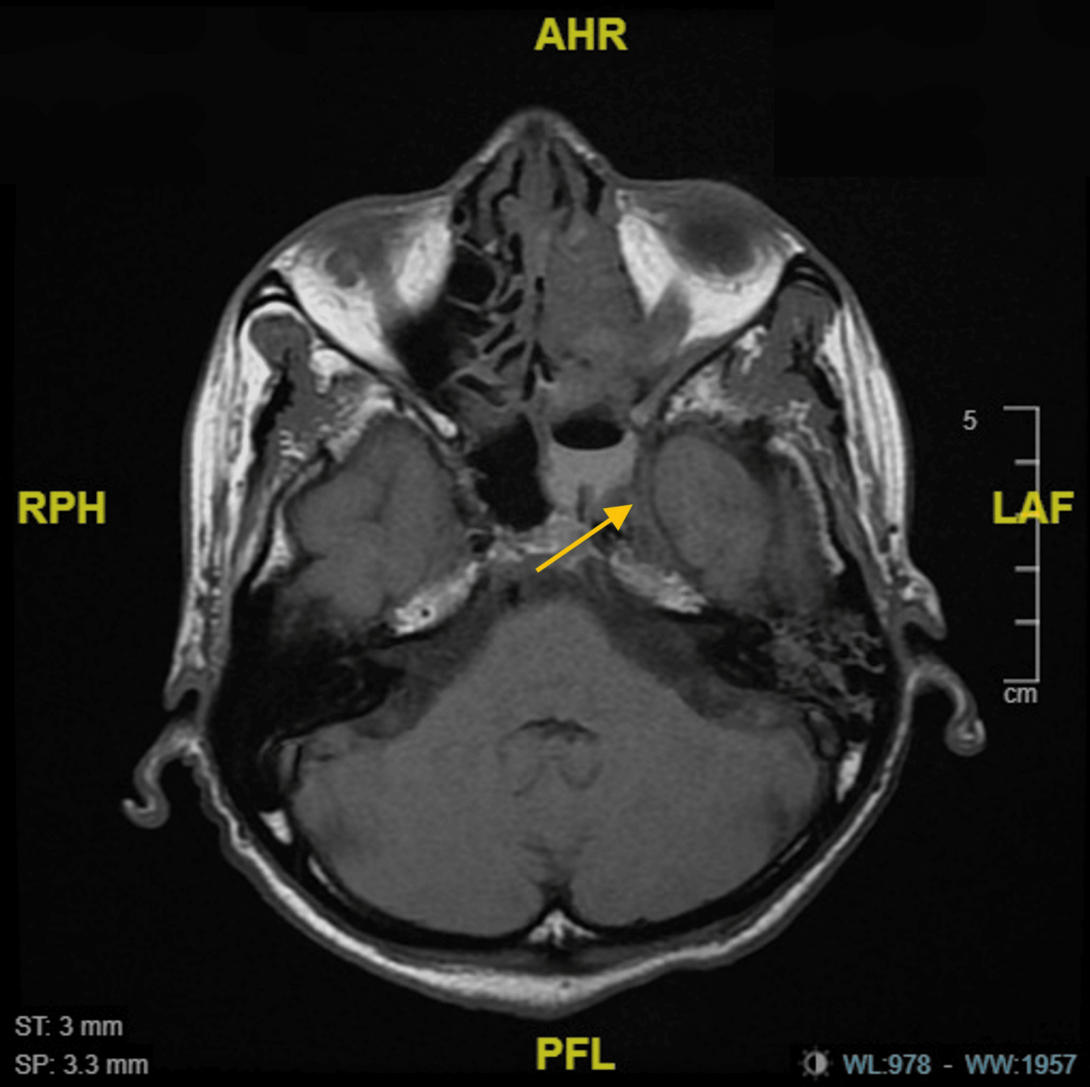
An axial (T1) MRI view showing the intracranial extension at the level of the fovea ethmoidalis (arrow).
